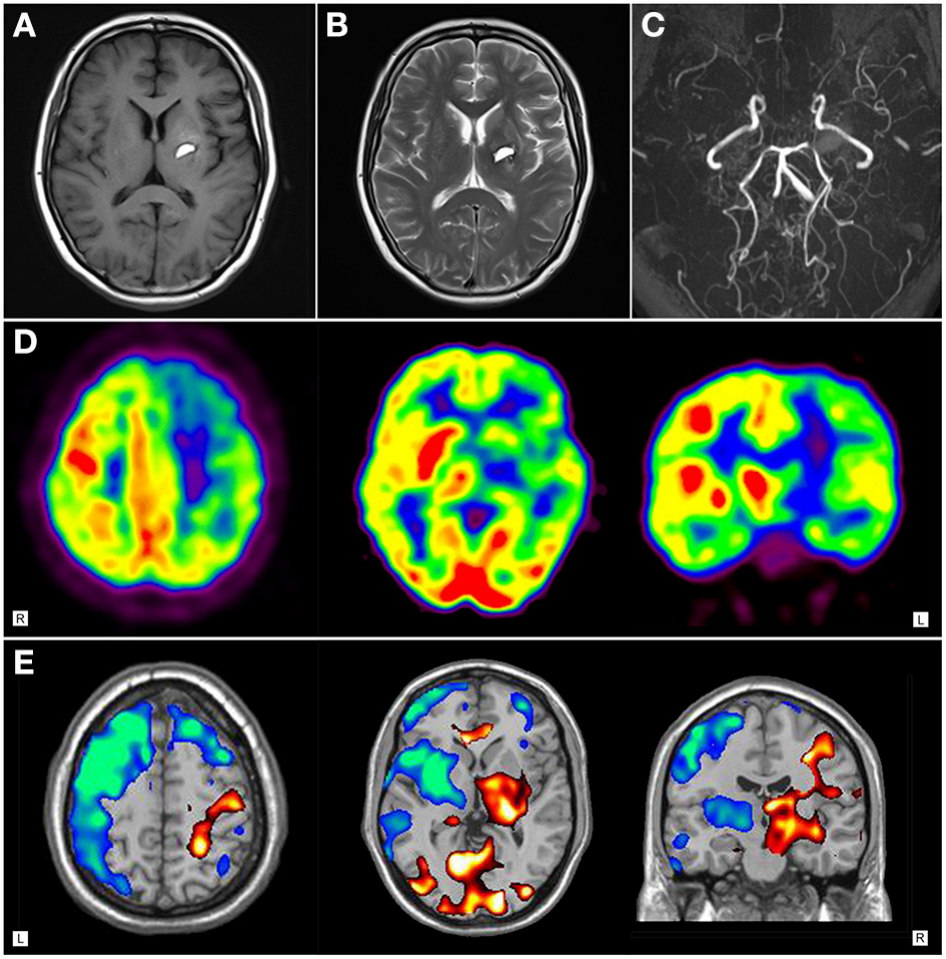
Neuroimaging findings of patient 2. (A)T1-weighted axial and (B) T2-weighted cranial magnetic resonance imaging showed hyperintense signal in the left basal ganglia. (C) Magnetic resonance cerebral angiography revealed severe stenosis of internal carotid arteries in the supraclinoid portion, with numerous collateral vessels. (D)
